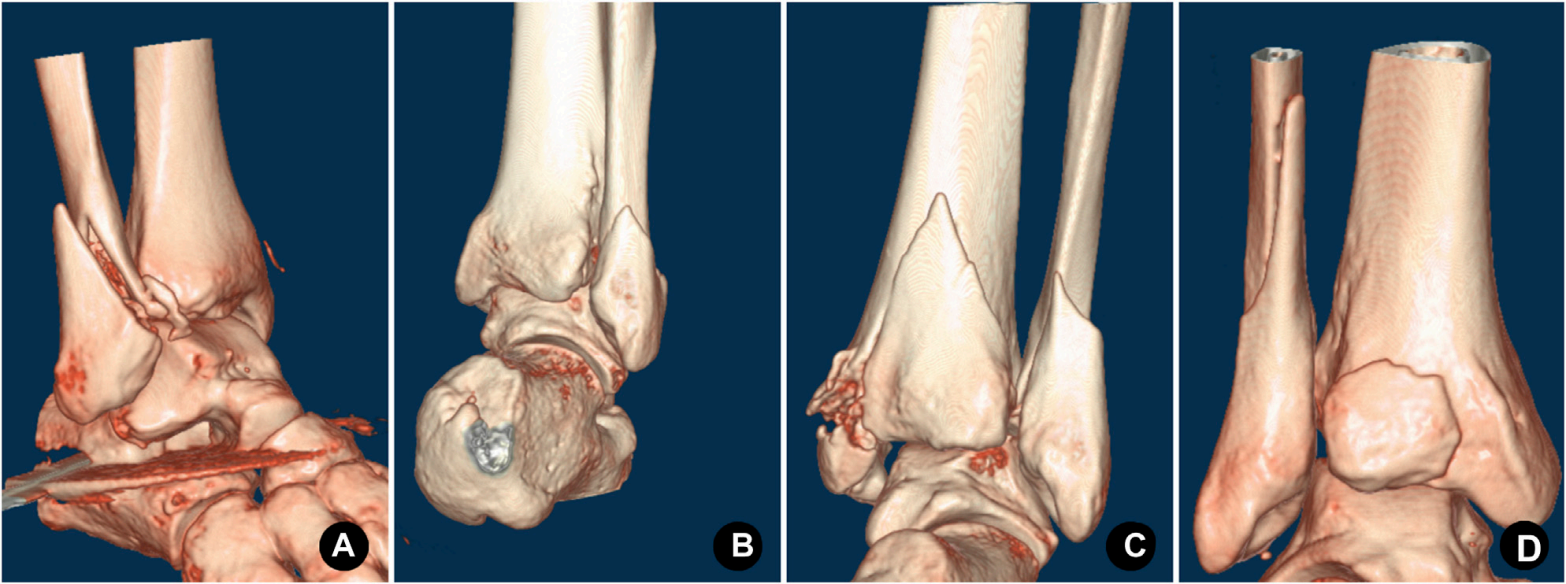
Distribution of the end-tip apex was classified into four zones in 3D-CT images. (A) Zone I, the apex located on the lateral ridge. (B) Zone II, the apex located on the posterolateral surface. (C) Zone III, the apex located on the posterior ridge. (D) Zone IV, the apex located on the medial surface.
